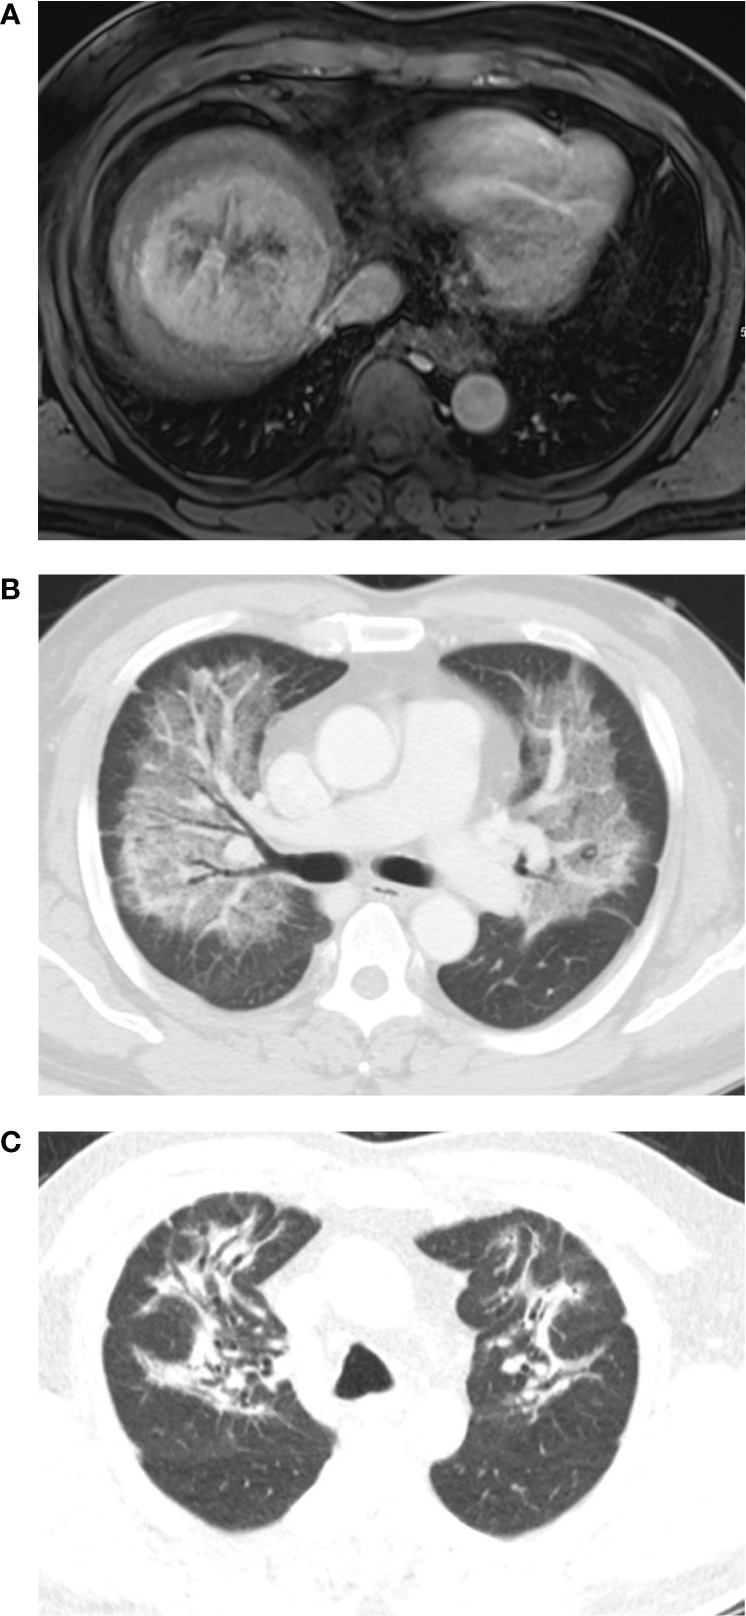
A 65-year-old man had a 9cm single mass in the right lobe. The lung shunt fraction was 14.1%, and 2.4 GBq of resin ^90^Y-microspheres were delivered into the right hepatic artery. The estimated lung dose was 21.01 Gy. (A) Magnetic resonance shows a mass in right lobe. (B) Chest CT scan 3.8 months after radioembolization shows diffuse consolidation and ground-glass opacity in both the lungs with subpleural sparing. (C) Chest CT scan 4 months after steroid therapy shows fibrotic changes in both the lungs.
